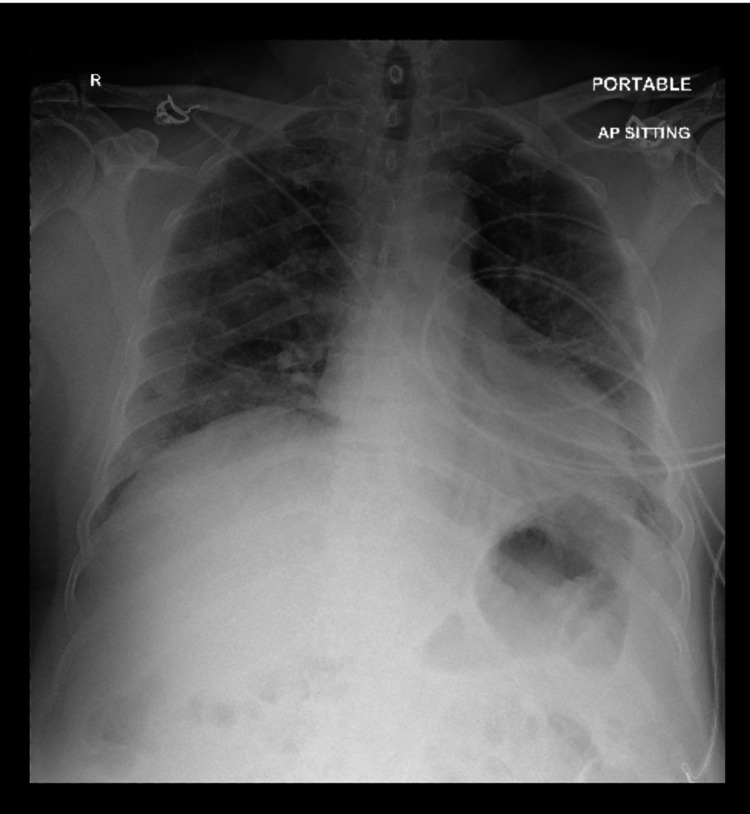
Chest X-Ray showing right middle and lower zone patchy airspace opacities without pleural effusion or pneumothorax
